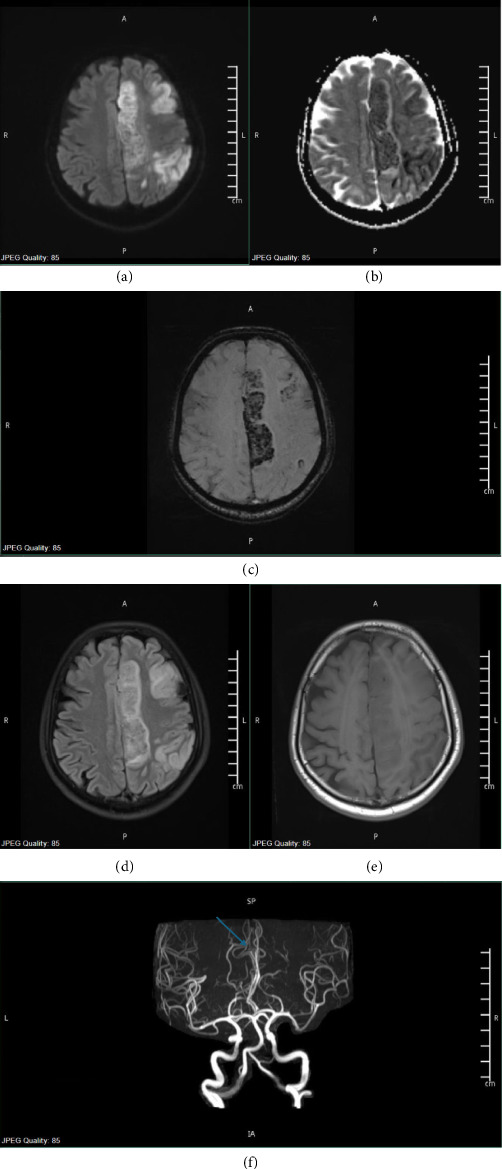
From left to right (a) and (b): This is a magnetic resonance imaging (MRI) axial diffusion-weighted long b value image (DWI) and apparent diffusion coefficient (ADC) map showing infarct in left anterior cerebral artery (ACA)-middle cerebral artery (MCA) territories. (c) This is an axial magnetic resonance imaging (MRI) susceptibility weighted image (SWI) showing extensive blooming artifact in the left parasagittal frontoparietal region consistent with hemorrhagic transformations. (d) T2 FLAIR sequence. (e) T1 sequence-extensive multifocal confluent parenchymal areas of diffusion restriction and T2/fluid attenuated inversion recovery (FLAIR) images showing hyperintense signal intensity in the left fronto-parieto-occipital cortical/subcortical, high left parasagittal frontoparietal regions. (f) 3D-time of flight (TOF) intracranial magnetic response angiography (MRA) showing attenuated caliber mid A2 segment of the left ACA (as compared to the right side) with no definite occlusion; however, there is asymmetric paucity of the distal branches.
